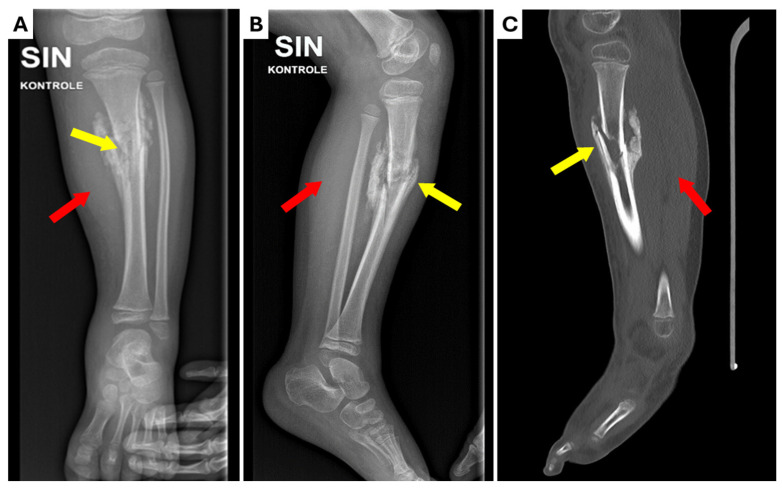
Radiological examinations performed at the Regional Hospital. (A) X-ray anterior–posterior projection; (B) X-ray lateral projection; (C) computed tomography (CT) scan of the lower left leg. Marked sclerotic hyperostosis in soft tissue (red arrows) around the fracture zone and non-union of tibial mid-diaphyseal fracture (yellow arrows) with moderate angular deformation (~15^o^) can be seen.
